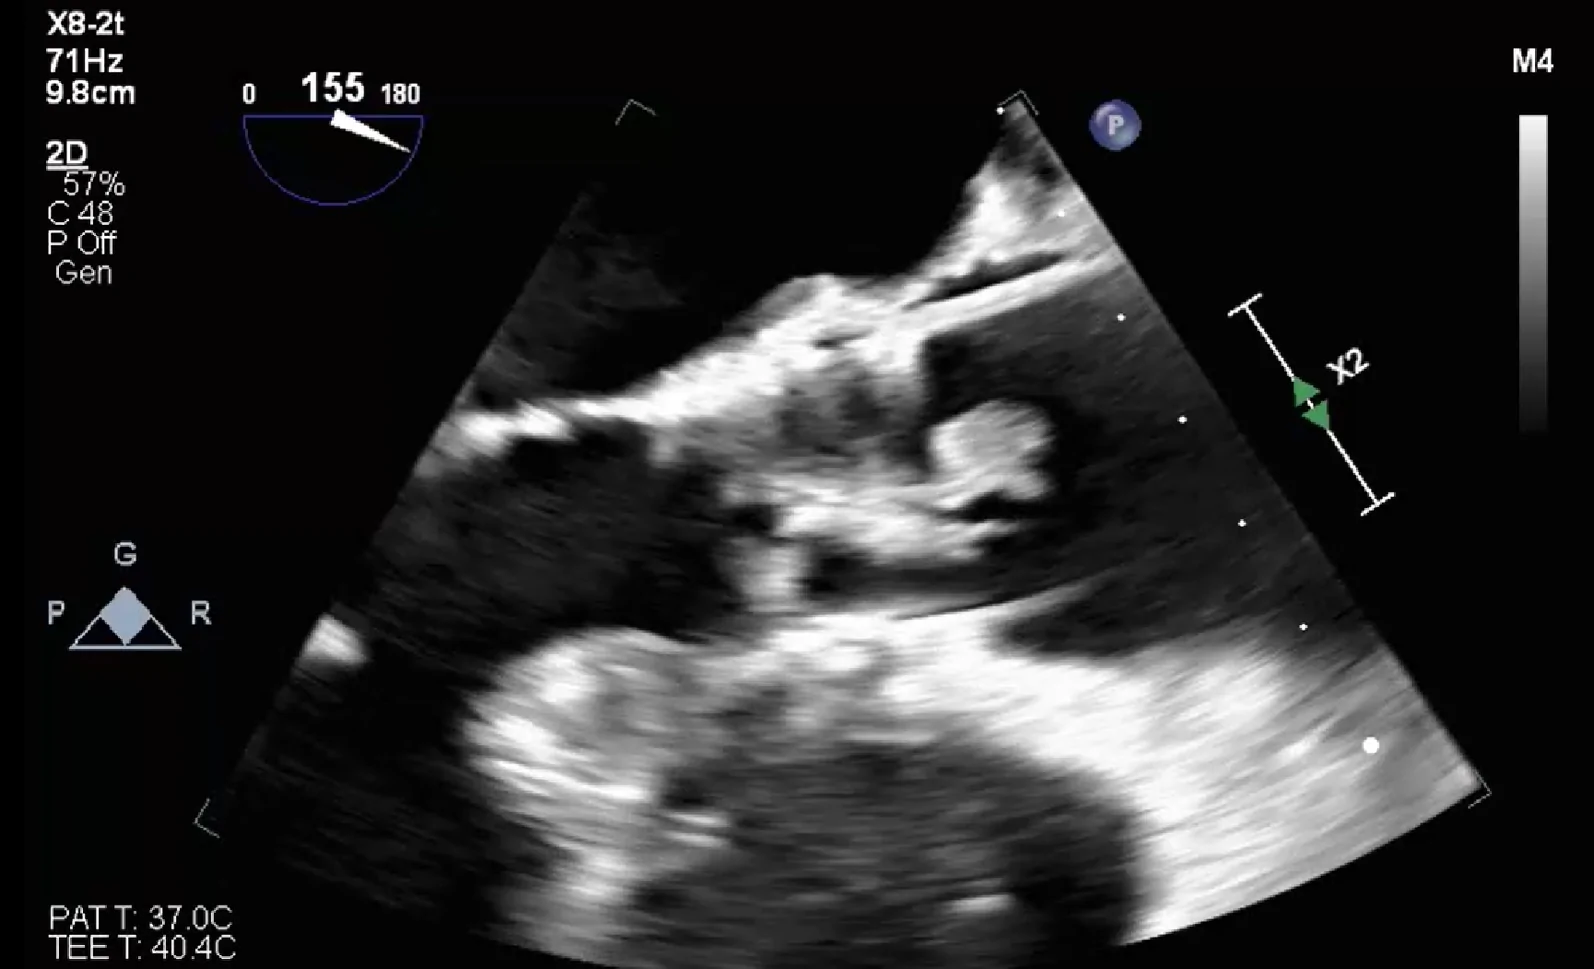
Preoperative transesophageal echocardiography demonstrated a large (about 25 mm), elongated, highly mobile vegetation attached to the transcatheter aortic valve prosthesis
